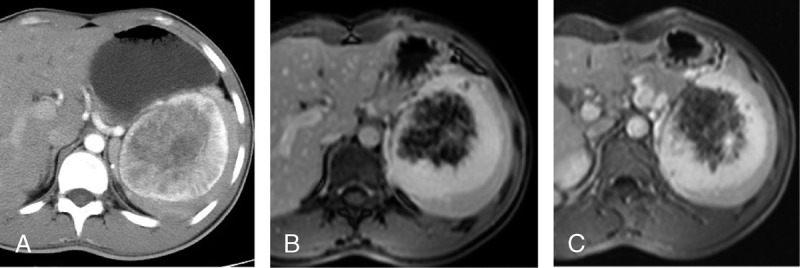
Sclerosing angiomatoid nodular transformation in a 21-year-old man. On the contrast-enhanced CT image (A), the lesion shows significantly enhancement with the pattern of spoke-wheel. On the images of contrast-enhanced MR (portal venous and delayed phase), the lesion shows a prominent enhancement with the pattern of progressive and centripetal, whereas no enhancement is seen in the center area (B, C).
